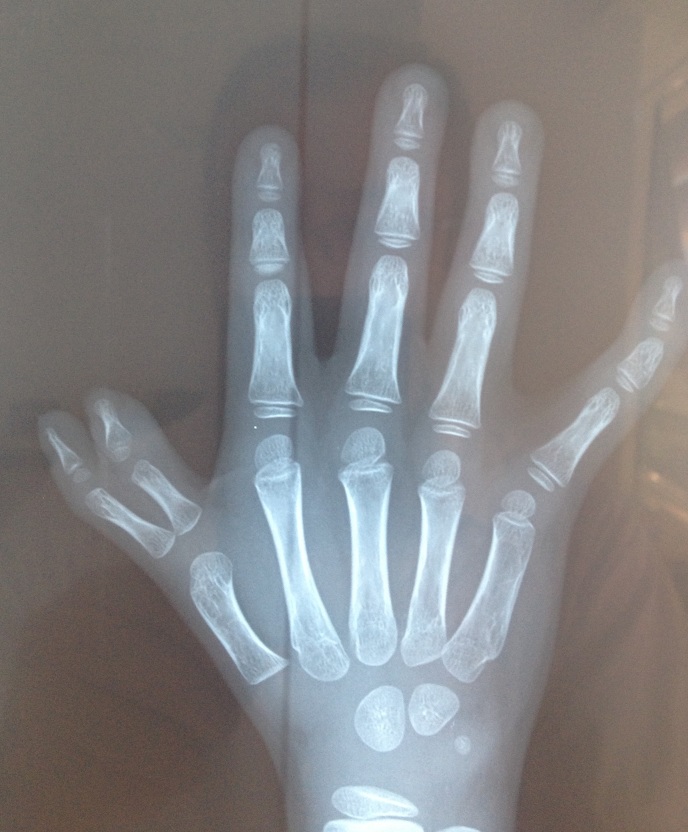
Radiographie de la main droite montrant une duplication du pouce type 4 selon la classification de Wassel
